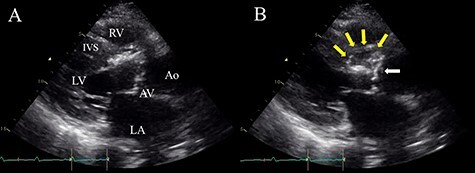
Preoperative TTE (parasternal long-axis view) showing vegetation on the aortic valve (white arrow) and aortic annular abscess extending to the IVS (yellow arrows); (A) end-systolic phase; (B) end-diastolic phase; LV, left ventricle; RV, right ventricle; LA, left atrium; AV, aortic valve; Ao, ascending aorta.
